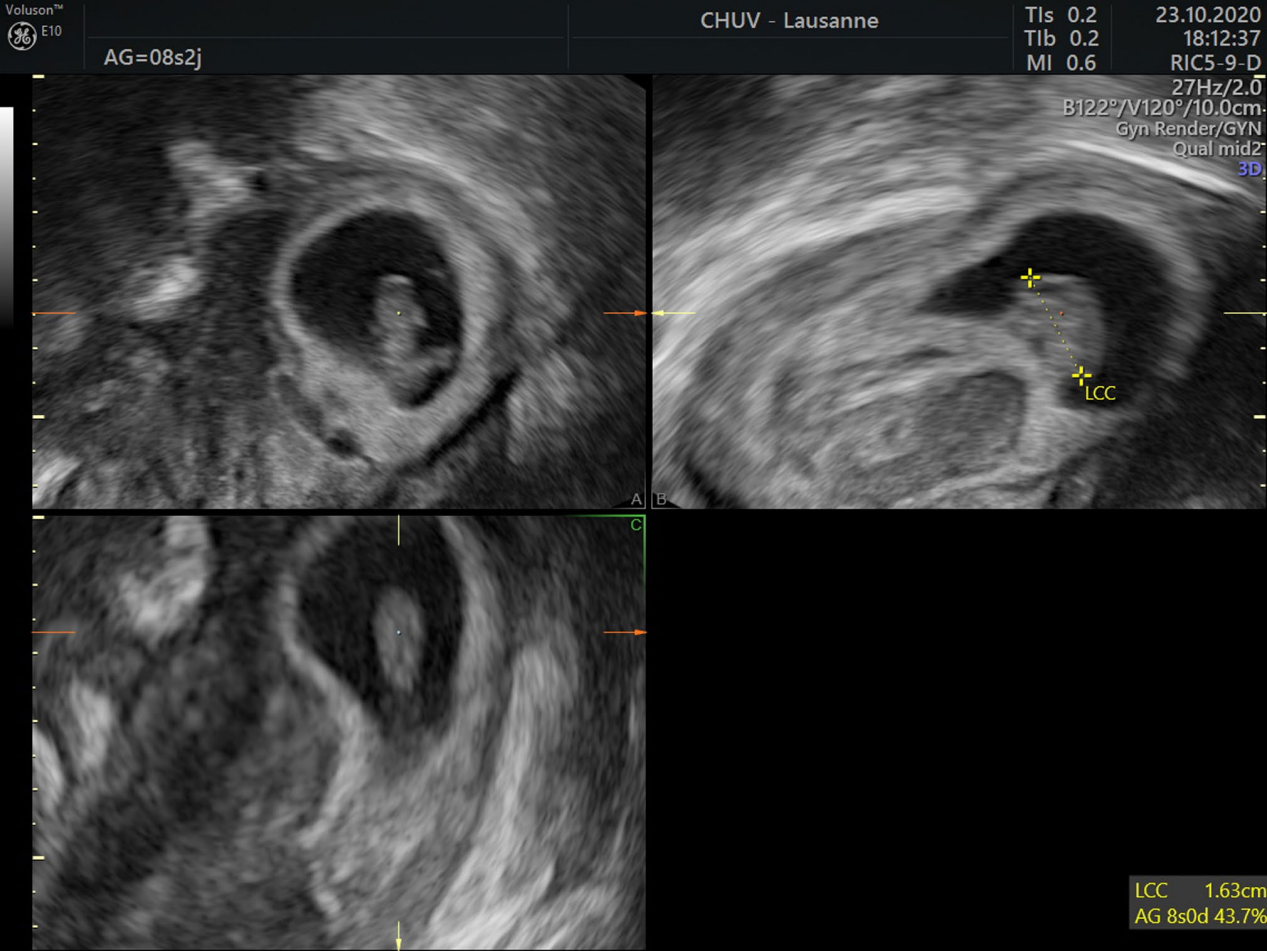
2D Ultrasound coronal view of angular pregnancy at 8wg
